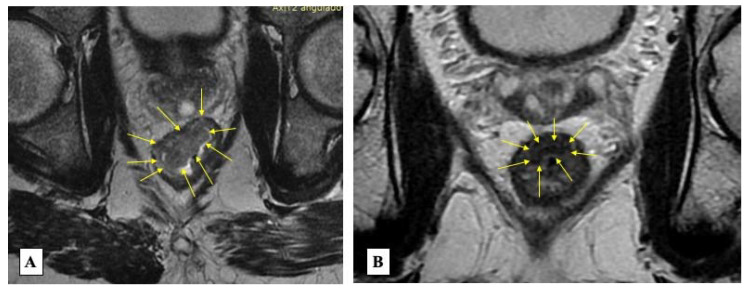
Radiological assessment using MR showing the baseline tumor (A—arrows) and an area of low-signal intensity areas consistent with a complete response in T2-weighted images (B—arrows).
